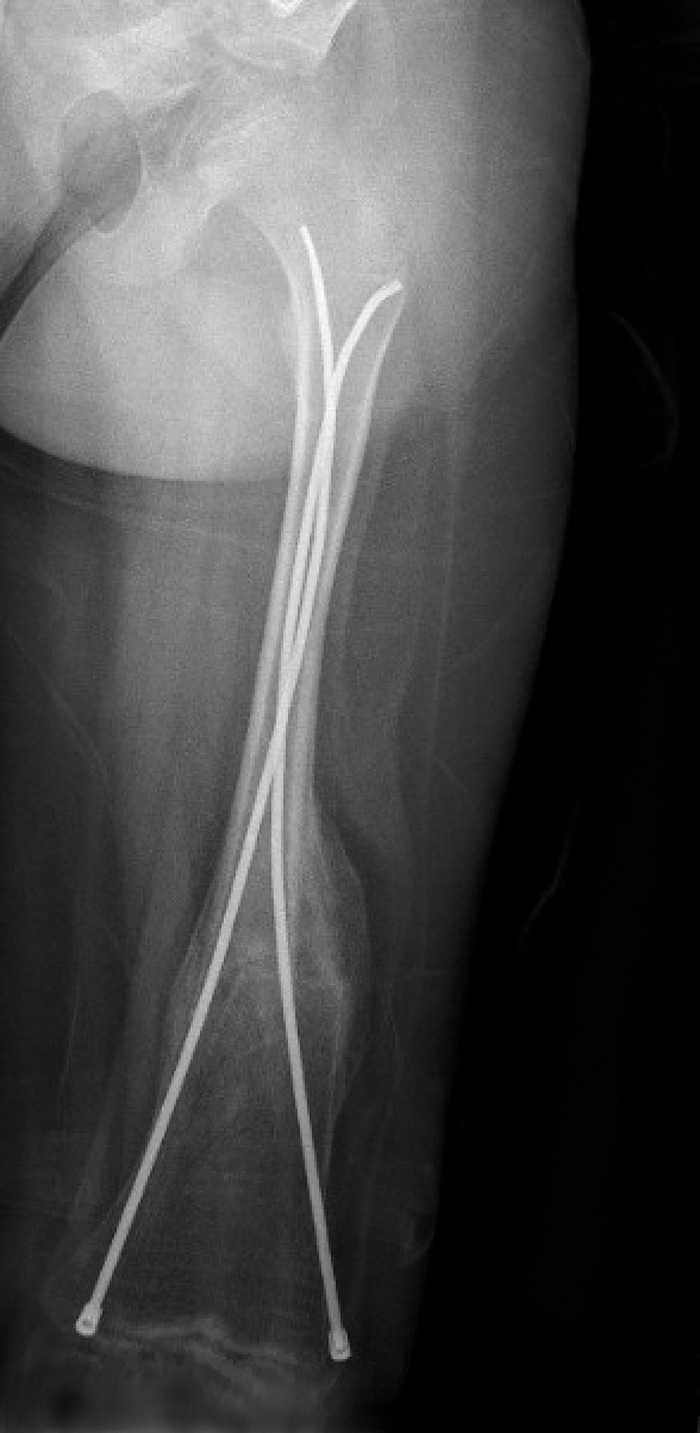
Radiograph of left femur of the male sibling showing a healed femur fracture after intramedullary nailing at age 4.
